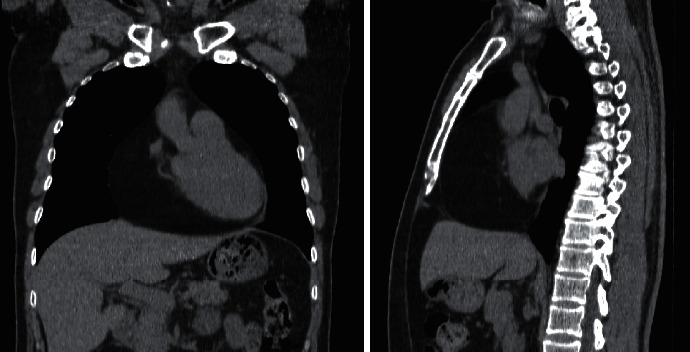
Voluminous pericardial mass. Pericardium tumor with a density similar to fat on a computed tomography scan. Note that the tumor causes posterior displacement of the heart and signs of a reduction in the dimensions of the right heart chambers.
